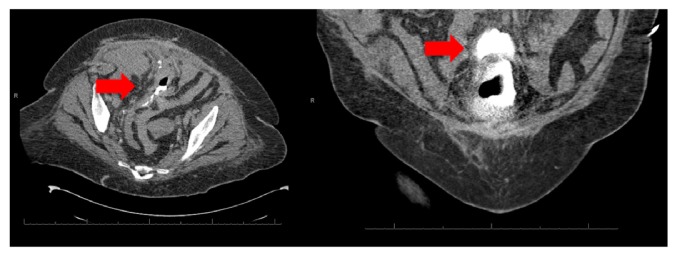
Contrast extravasation demonstrated with computed tomography cystogram (arrow).
